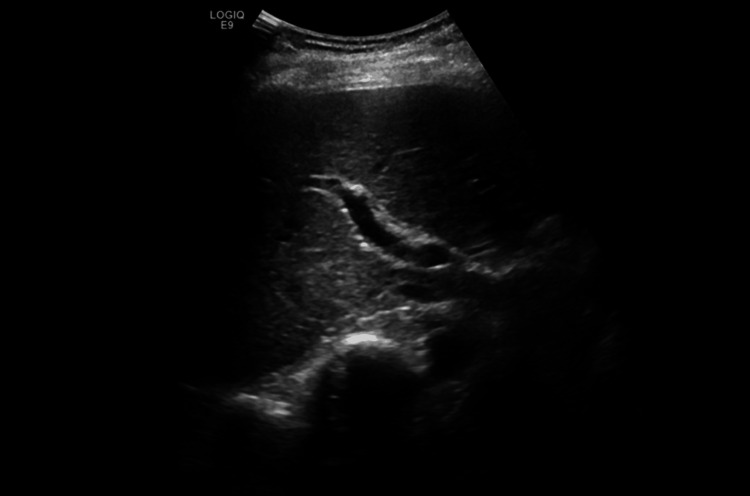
Ultrasound of the liver shows normal parenchyma and no biliary duct dilation
